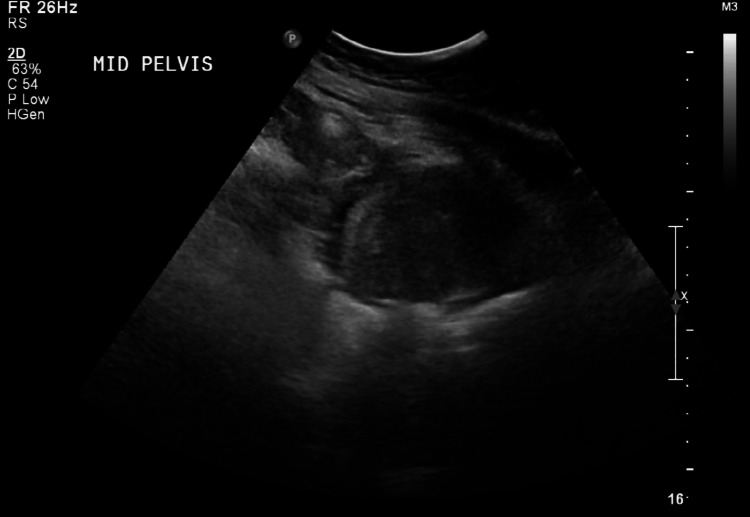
Transabdominal ultrasonography of the pelvis showing a small left adnexal collection
